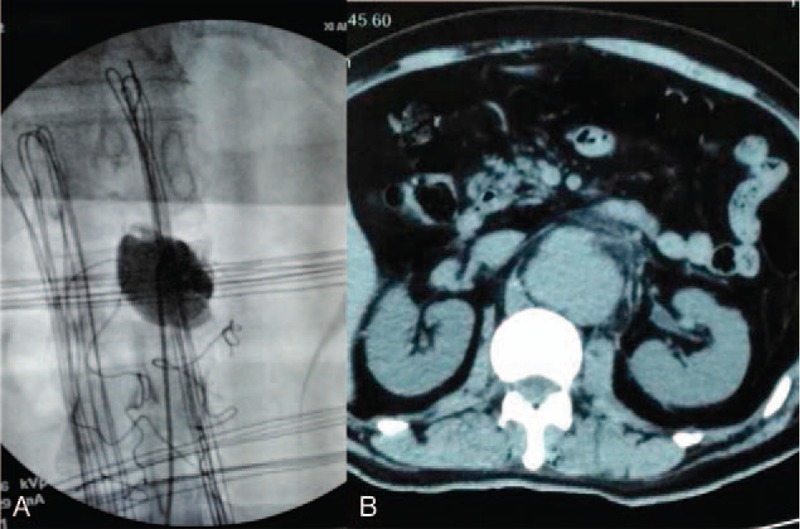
Male, 55 years old, L2 vertebral fracture (type A1): intraoperative angiography revealed abdominal aortic injury (A); postoperative CT showed pseudoaneurysm formation (B). CT = computed tomography.
